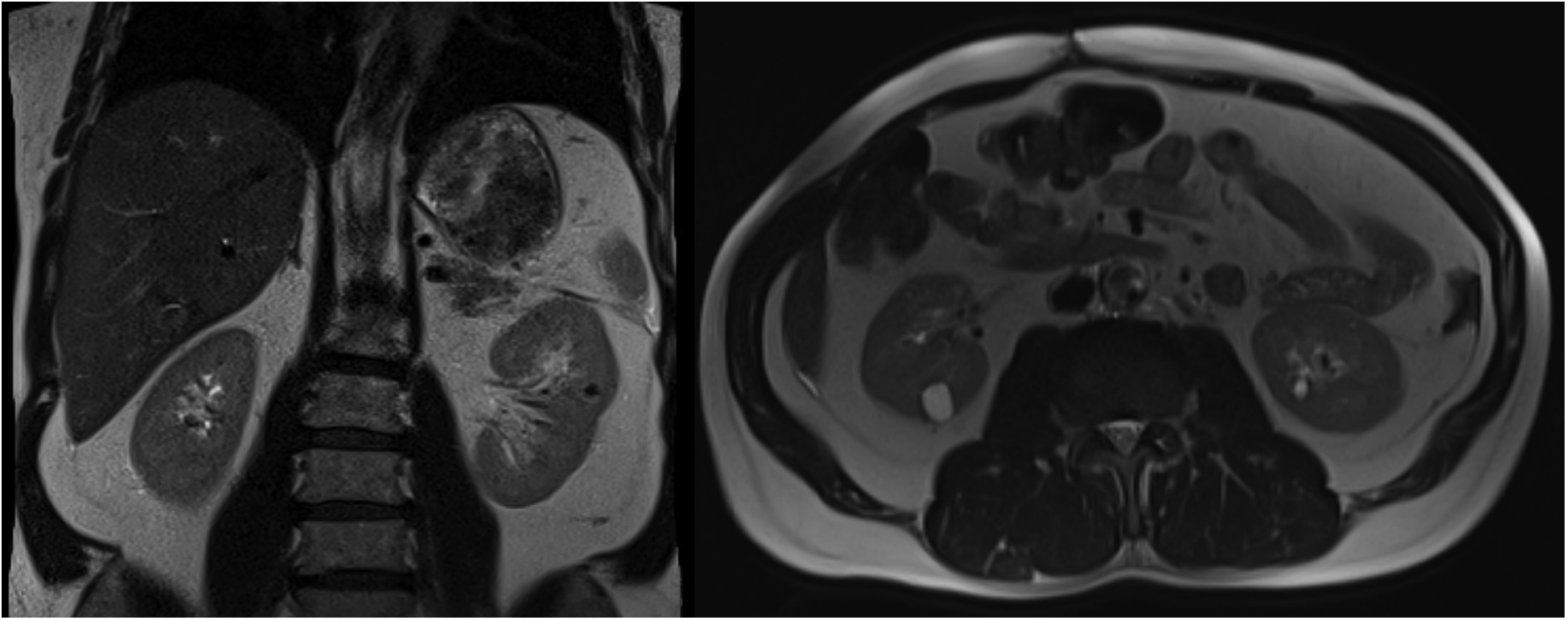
Magnetic resonance images at 1 year postoperatively showing successful resection of a giant cystic pheochromocytoma.
